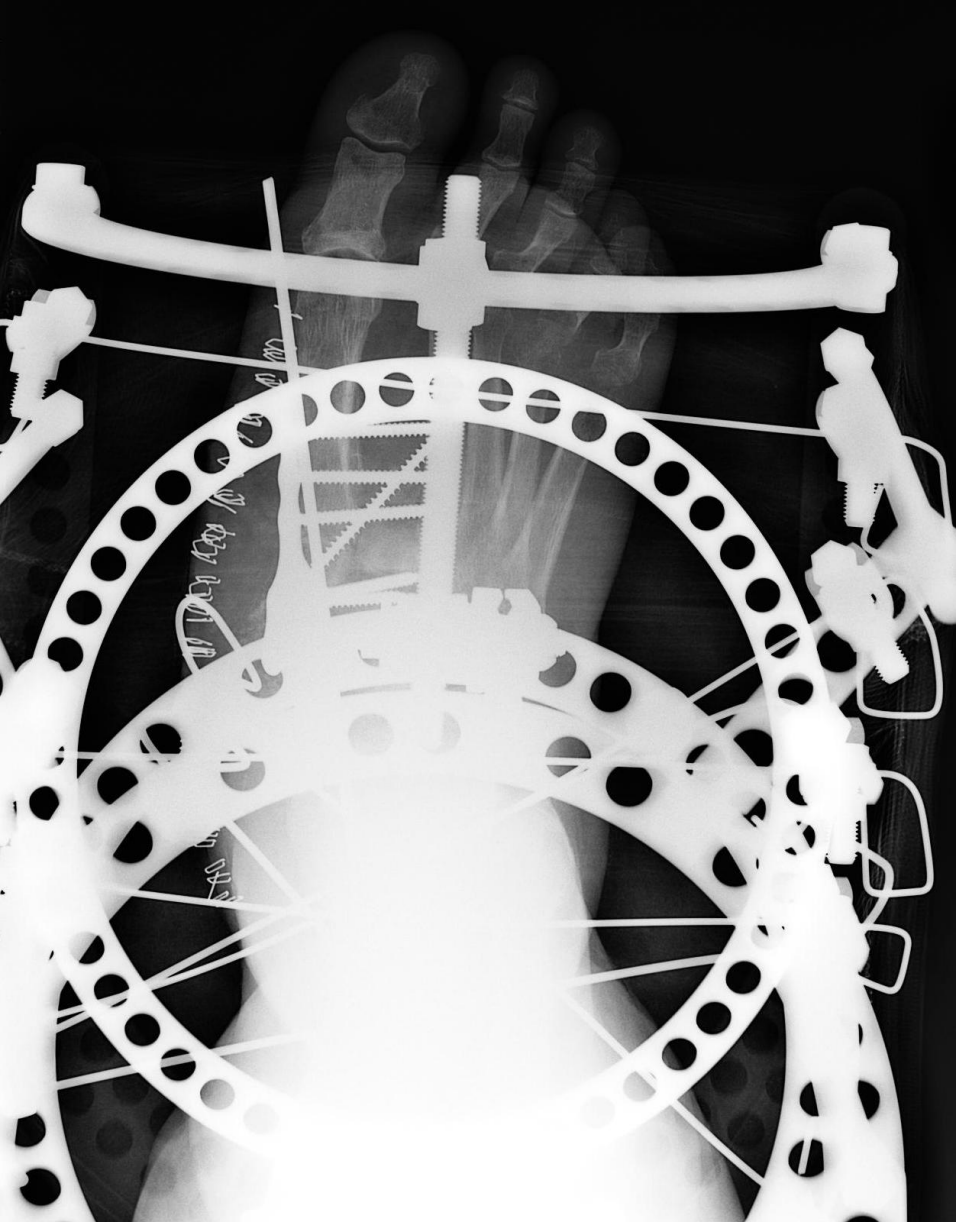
Postoperative anteroposterior radiographic view showing the multiple midfoot arthrodesis sites with combined internal and external fixation methods.
